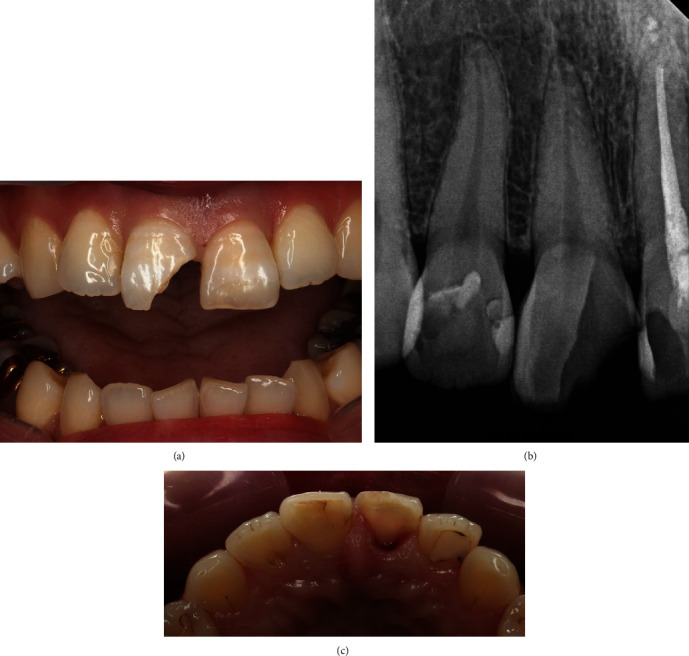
(a) Intra-oral photograph (Buccal), (b) Periapical view, (c) Intra-oral photograph (Occlusal) at first visit.
